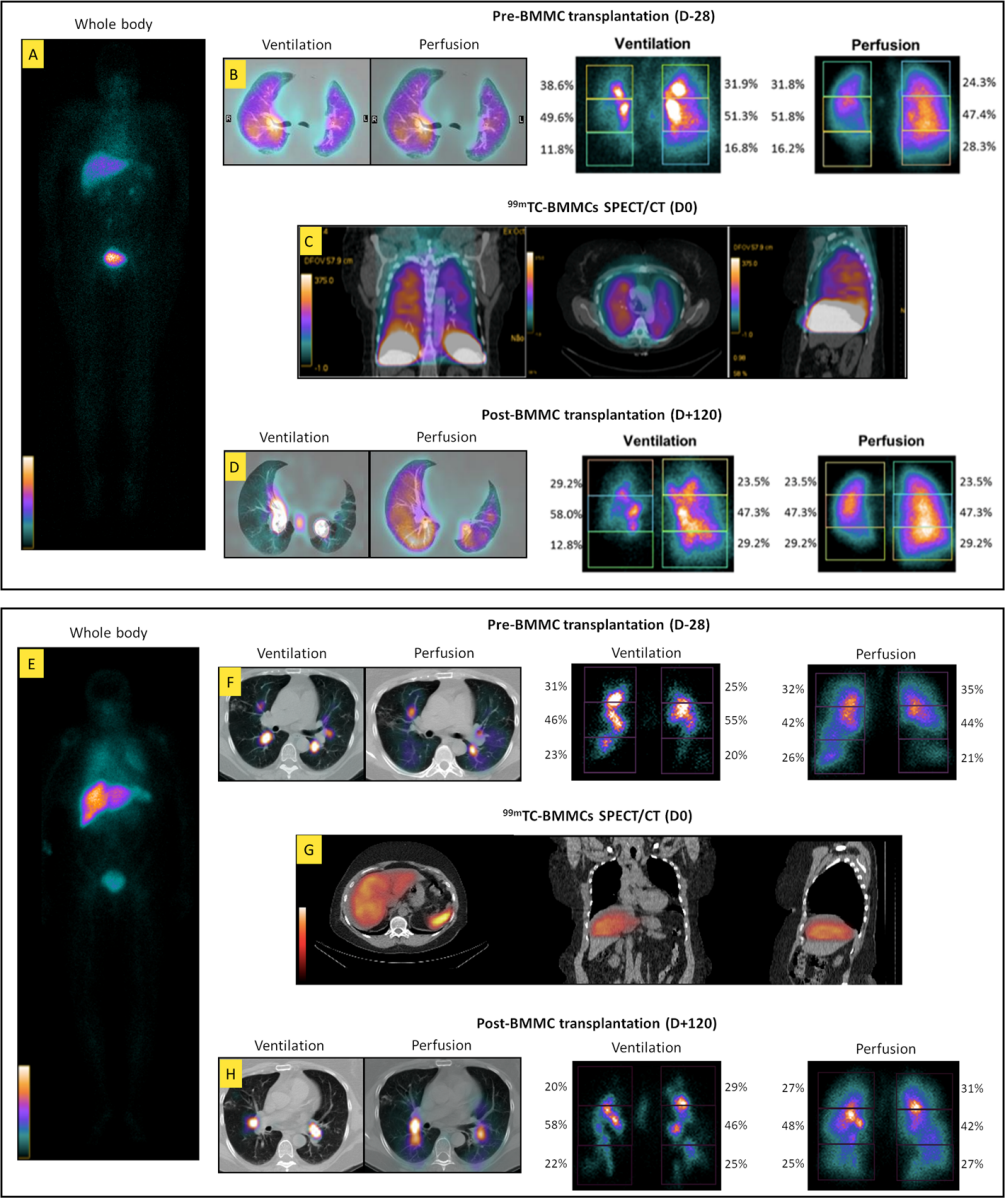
Imaging of patients 1 (upper panel) and 2 (lower panel). a, e Whole-body (WB) scintigraphy 2 h after intravenous administration of ^99m^Tc-BMMCs. Uptake found in the liver, lungs, heart, blood pool, kidney, and bladder. b, f Lung ventilation/perfusion SPECT/CT 28 days before BMMC transplantation. c, g^99m^Tc-BMMC SPECT/CT demonstrating liver and lung uptake 2 h after BMMC transplantation. d, h Lung ventilation/perfusion SPECT/CT 120 days after BMMC transplantation
